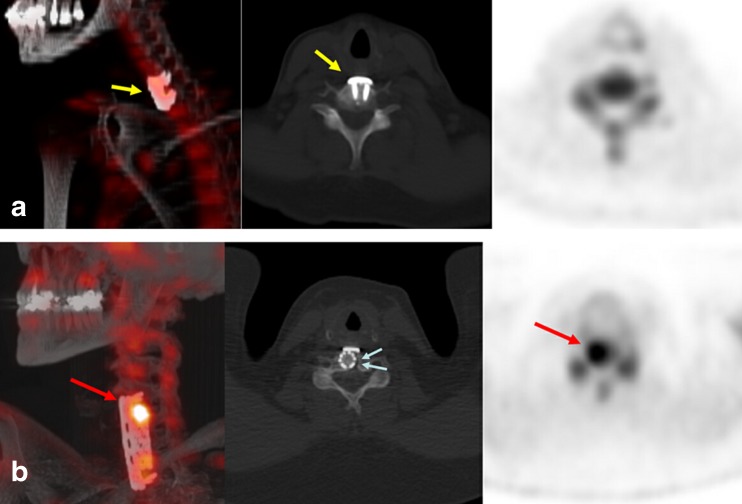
Comparison of patient 5 and patient 17. a Patient 5: oblique 3-D PET/CT fusion maximal intensity projection image, axial noncontrast CT image, and axial coregistered PET image show metallic spinal fixation hardware in the cervical neck (*yellow arrows*). There is no evidence of abnormalities on the PET image or the CT image. The patient was therefore referred for conservative management and had eventual improvement of symptoms. b Patient 17: oblique 3-D PET/CT fusion maximal intensity projection image, axial noncontrast CT, and axial coregistered PET image show metallic hardware in the cervical neck. An abnormally intense focus is apparent in the proximal spinal fixation cage (*red arrows*), and very subtle radiolucency around the metallic fixation cage (*blue arrows*) is apparent on the CT image. The patient was referred for surgical exploration and hardware loosening and abnormal spinal mobility was confirmed in this region
